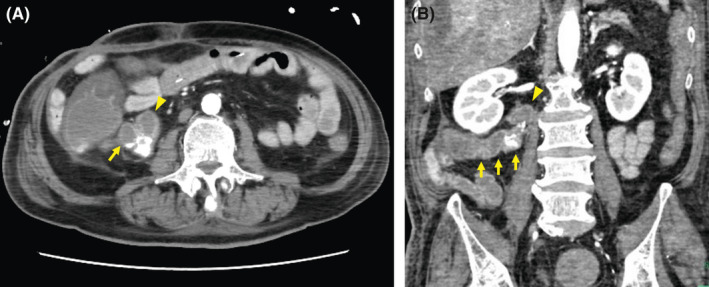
(A) Abdominal contrast‐enhanced computed tomography shows extravasation of contrast medium in the cecum (arrow) and duodenum (arrowhead). (B) The coronal view shows the cecum deforming horizontally (arrows) and in close contact with the duodenum (arrowhead)
